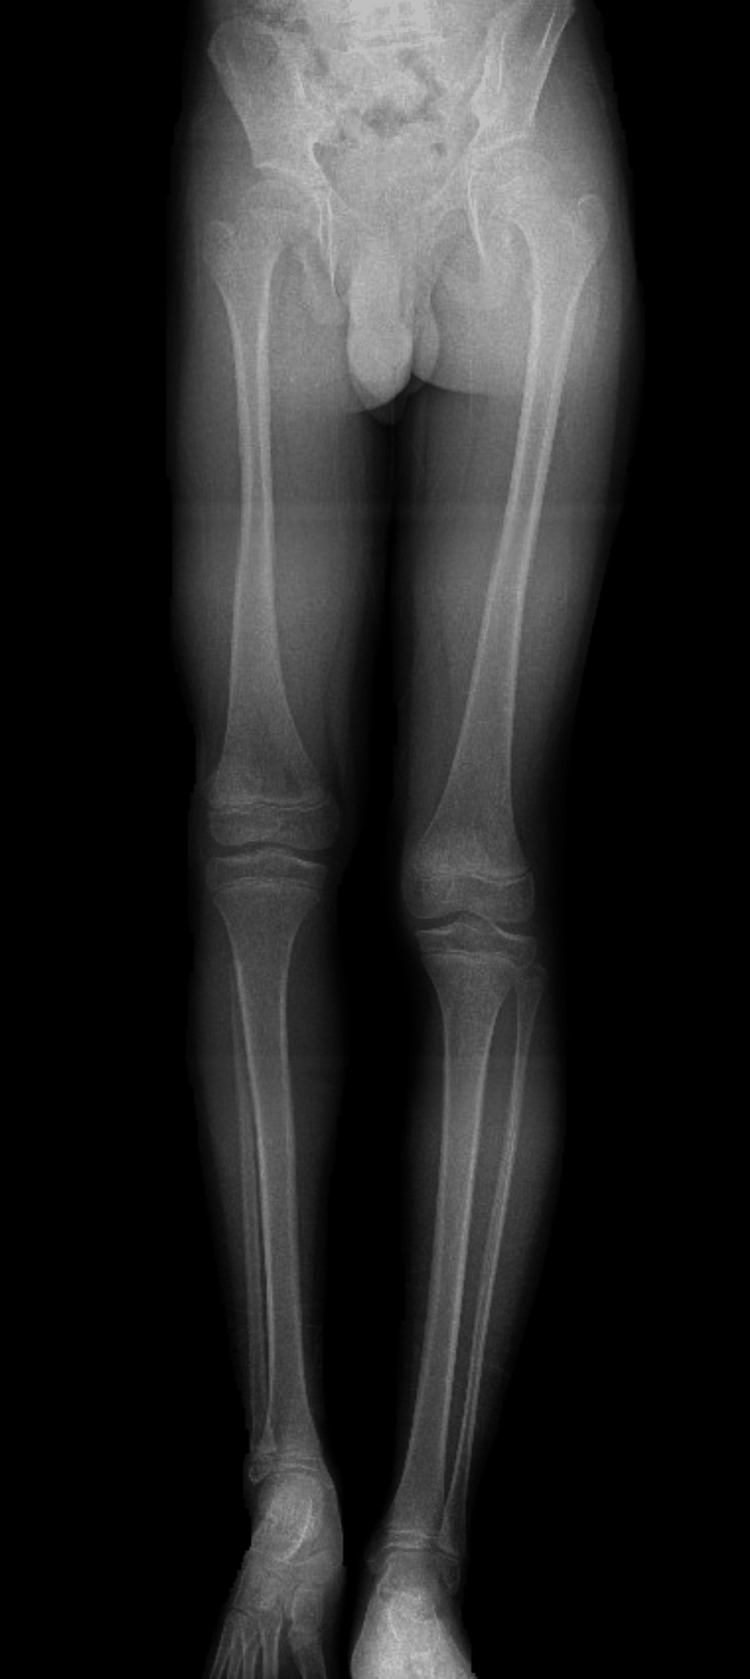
Long leg radiograph revealing right femoral hypoplasia with the absence of fibular head
